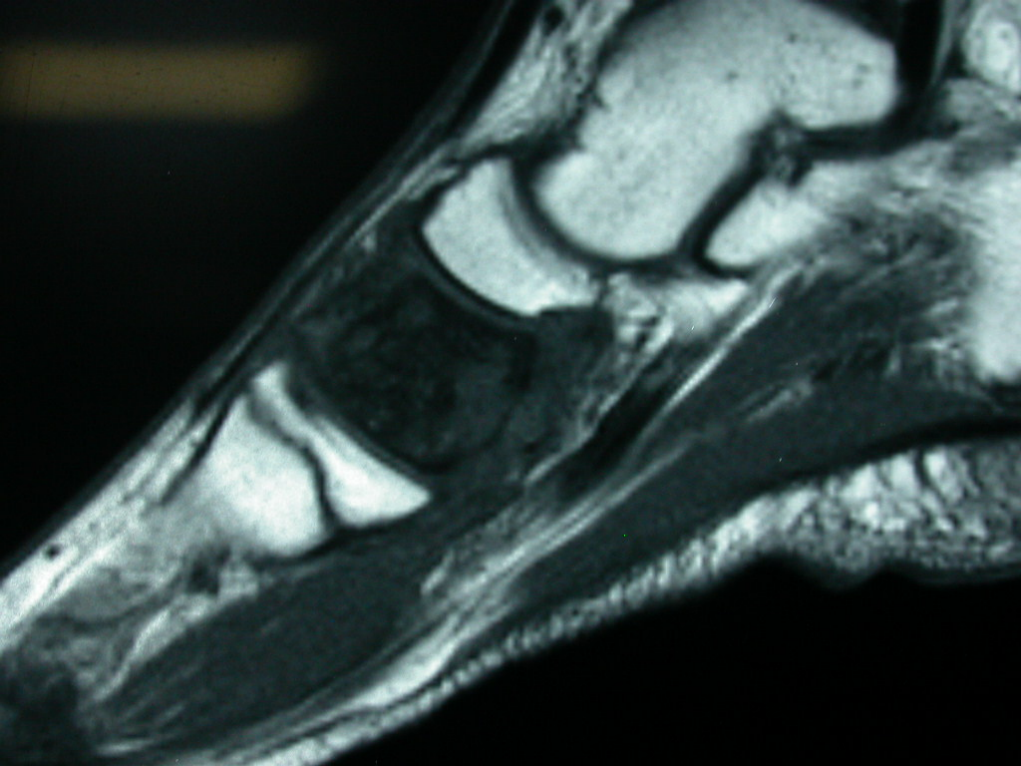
MRI of 1b, showing signal changes in whole of medial cunieform.
